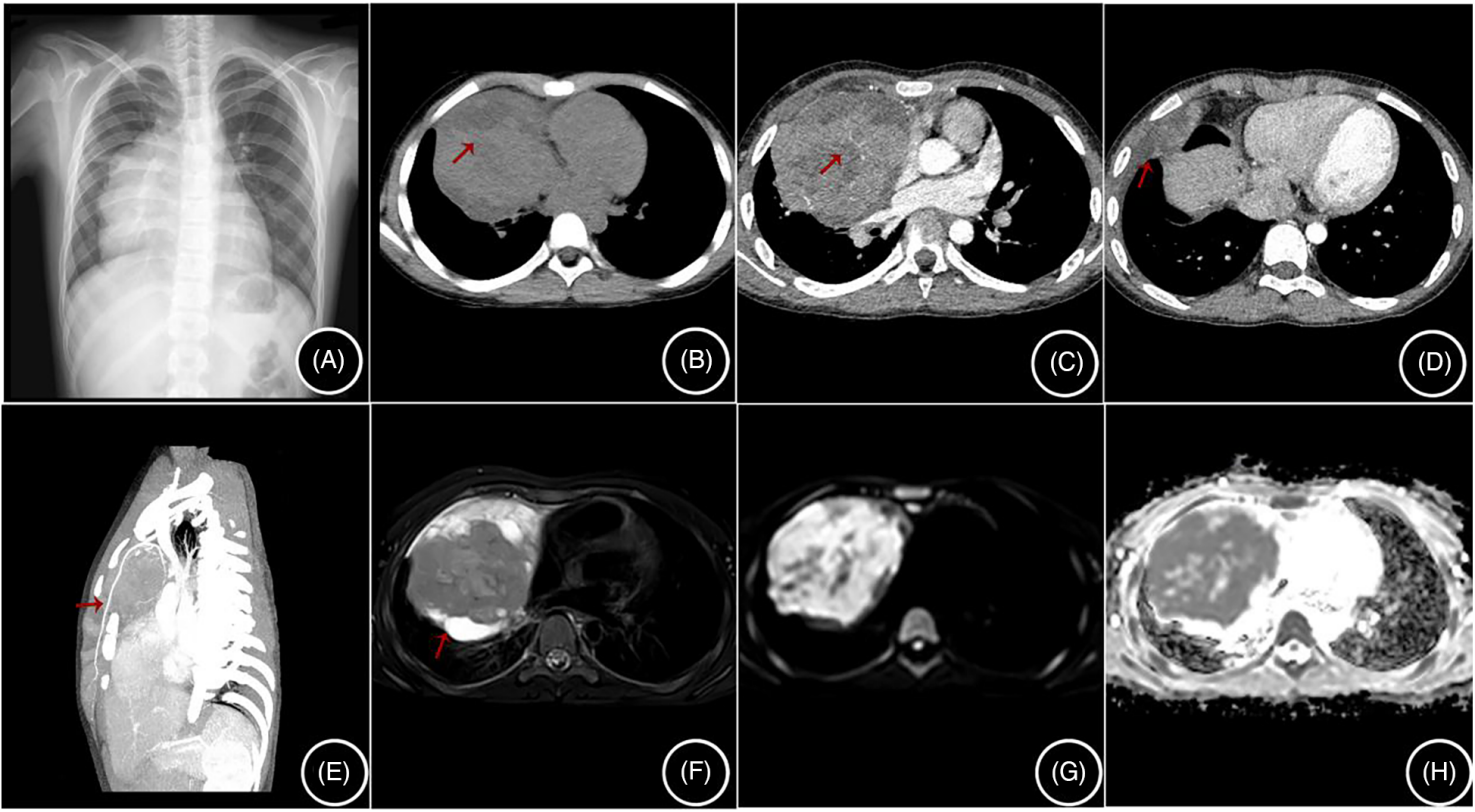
(A–H) are from the same case: (A) shows a mediastinal mass on a chest radiograph; (B) shows cystic necrosis (arrow) on a non-contrast CT scan; (C) shows tortuous small vessels within the enhanced lesion (arrow); (D) shows invasion of the right pleura (arrow); (E) shows the lesion being supplied by branches of the internal thoracic artery; (F) shows mixed signals in the lesion on MRI T2 fat-saturated images, with cysts showing high signal; and (G,H) show high signal in the solid components of the lesion on DWI and low signal on ADC.
